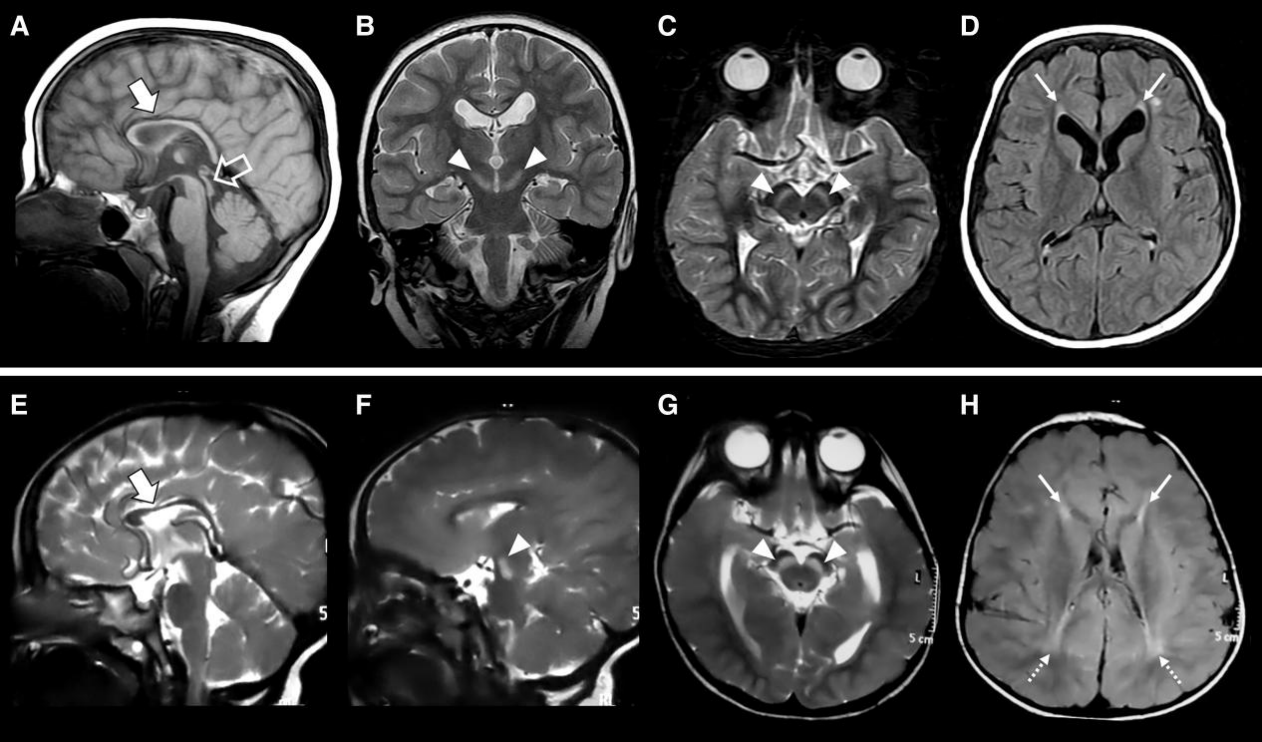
Neuroimaging features of LNPK-related disorder. Brain MRI studies performed in Patient II:1 from Family 6 at 4 years of age (A–D) and in Patient II:2 from Family 7 at 2.5 years of age (E–H). Sagittal T_1_- (A) or T_2_-weighted (E) images demonstrate corpus callosum hypoplasia with prevalent involvement of the anterior portions (thick arrows). Coronal (B), axial (C, G) and sagittal (F) T_2_-weighted images reveal symmetric marked T_2_ hyperintensity of the substantia nigra (arrowheads). Note the ‘ears-of-the-lynx’ sign (thin arrows) on axial FLAIR images (D, H) consisting of hyperintense signal of the forceps minor bilaterally, which resembles the shape of the ears of a lynx with their characteristic apical hair tuft. Additional posterior periventricular white matter signal alterations are noted in Patient II:2 from Family 7 (dotted arrows). A short midbrain is also visible in Patient II:1 from Family 6 (empty arrow).
